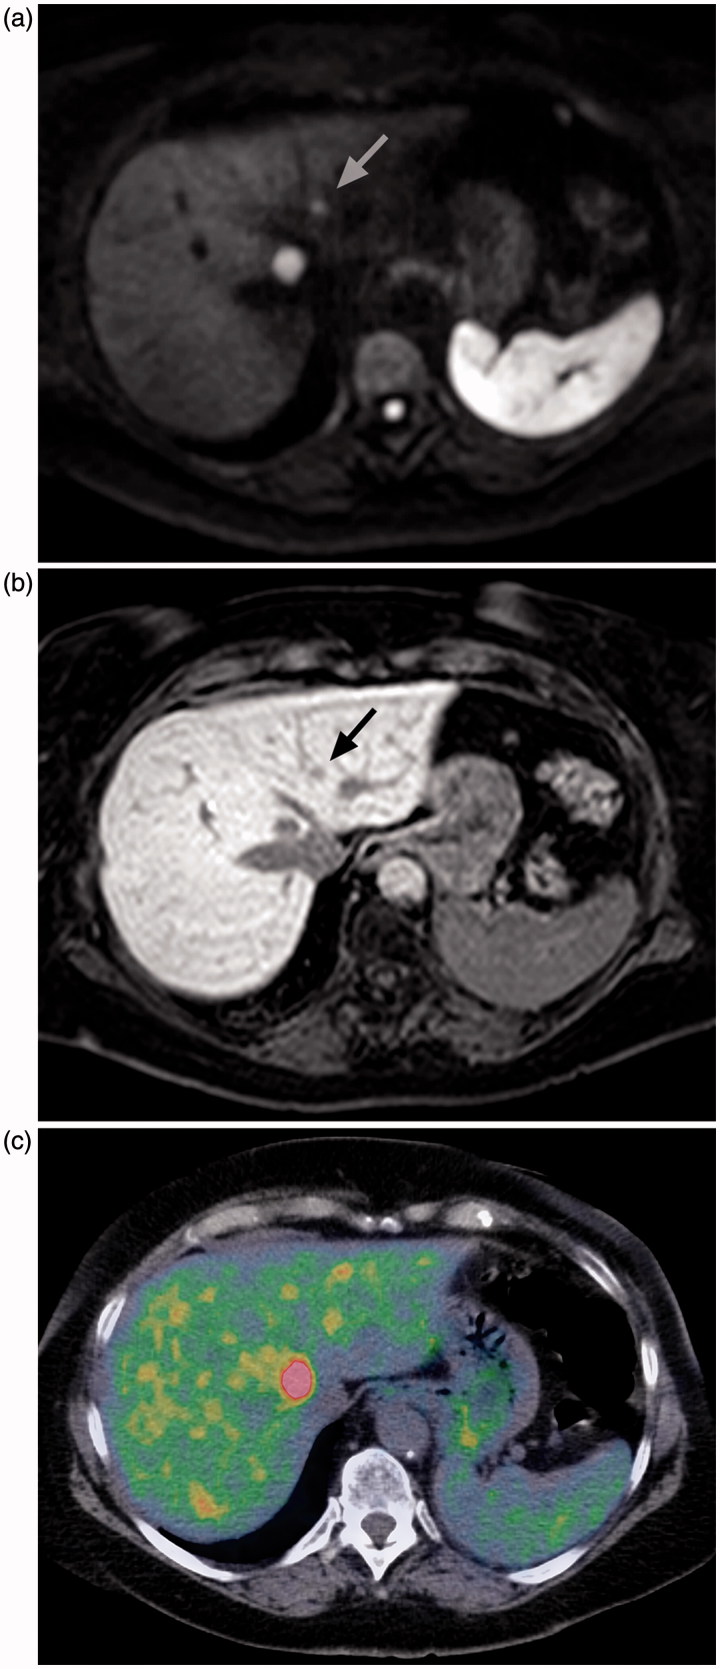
Axial diffusion-weighted MRI (a), contrast-enhanced MRI, hepato-biliary phase (b), and 5-HTP PET/CT (c) in the same patient. The larger lesion is clearly visible in all three, but the smaller one (indicated with arrow in MR images) is difficult to separate from the background uptake on the PET/CT image.
